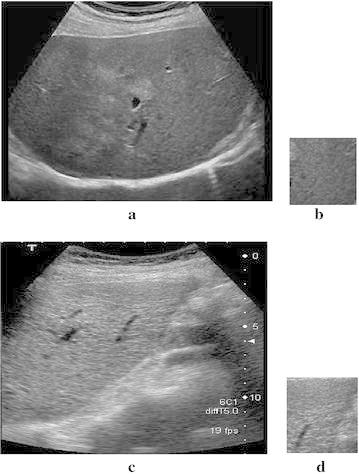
a Ultrasound image of normal liver, b ROI defined by radiologist from normal liver, c ultrasound image of cirrhosis liver, d ROI defined by radiologist from cirrhosis liver
